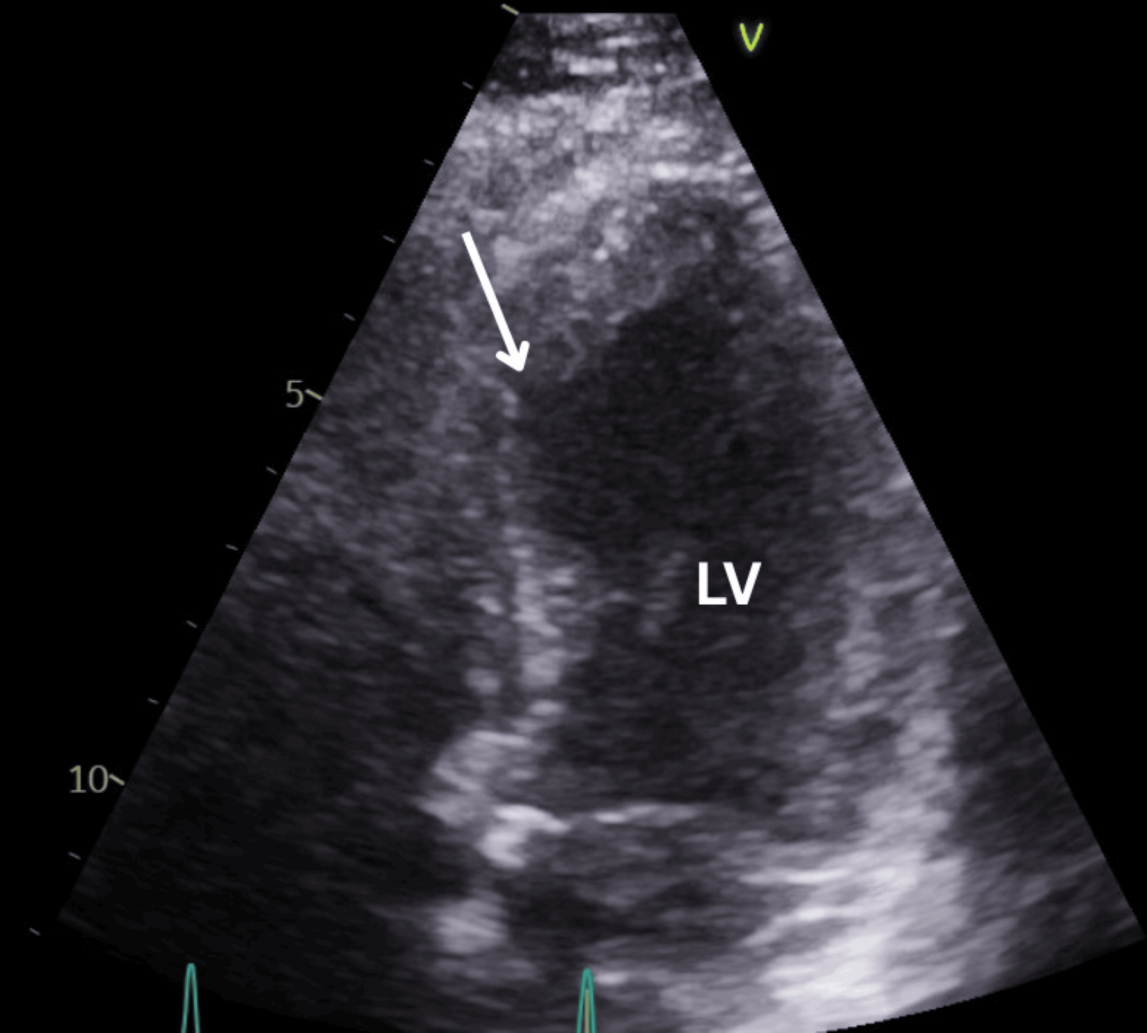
Transthoracic echocardiogram (systolic phase) showing the left ventricle (LV) with mid-septal ballooning, whereas the apex has normal contraction
